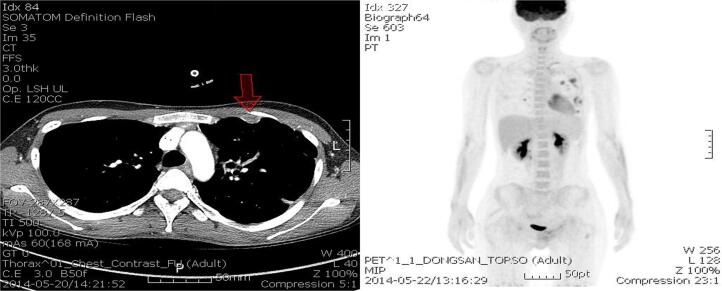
(a) HRCT shows various sized multiple nodules at lungs, fissure, and pleura at both hemithorax. (b) PET CT shows partial resolution of vulvar lesion, complete resolution of pelvic bone metastases and aggravation of multiple lung and left pleura.
